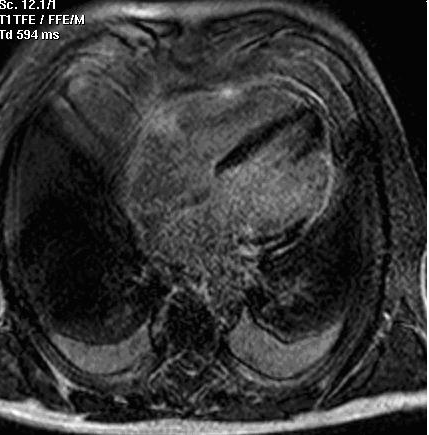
Cardiac MRI after gadolinium injection demonstrated a transmural area of DE in the lateral wall of the left ventricle. A bilateral pleural effusion was also evident.
